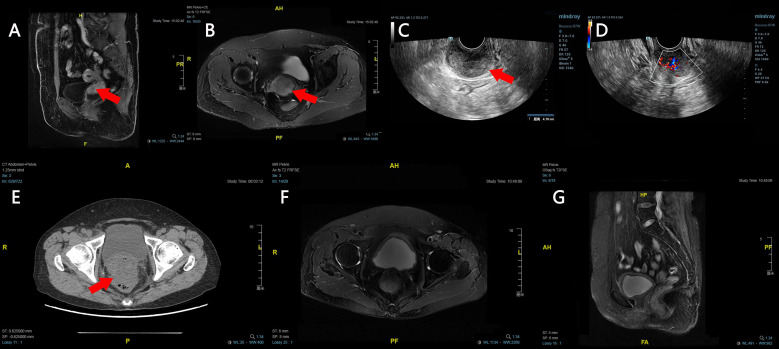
Imaging findings before and after treatment for cervical sarcoma. (A, B) Preoperative pelvic magnetic resonance imaging demonstrates a solid mass (red arrows) within the cervix. (C, D) Preoperative transvaginal ultrasound reveals a solid cervical mass measuring 4.7 cm × 4.2 cm × 2.8 cm (red arrow), with rich internal blood flow signals on color Doppler. (E) A pelvic computed tomography scan obtained two months postoperatively showed a new mass (red arrow, 5.22 cm × 4.8 cm) located between the bladder and rectum, indicating disease progression. (F, G) Pelvic magnetic resonance imaging after six cycles of immunotherapy shows no evidence of a residual or recurrent mass in the pelvic cavity. (A) sagittal LAVA Flex T1 fat-suppressed contrast-enhanced image; (B) axial FS T2 FRFSE sequence; (F) axial FS T2 FRFSE sequence; (G) sagittal FS T2 FSE sequence.
